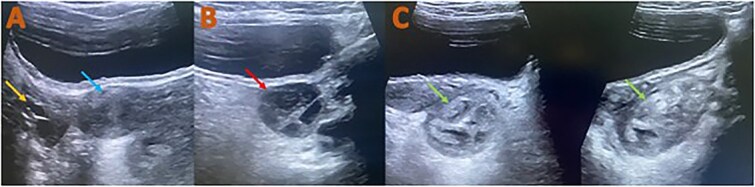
Ultrasound of the pelvis: A) shows the normal size and texture of the right ovary and normal uterus. B) Shows normal size and texture of the left ovary. C) shows left adnexal well defined echogenic mass lesion showing internal heterogenous texture measured (38 × 40 × 50mm = 40 cc) and avascular on color doppler US.
